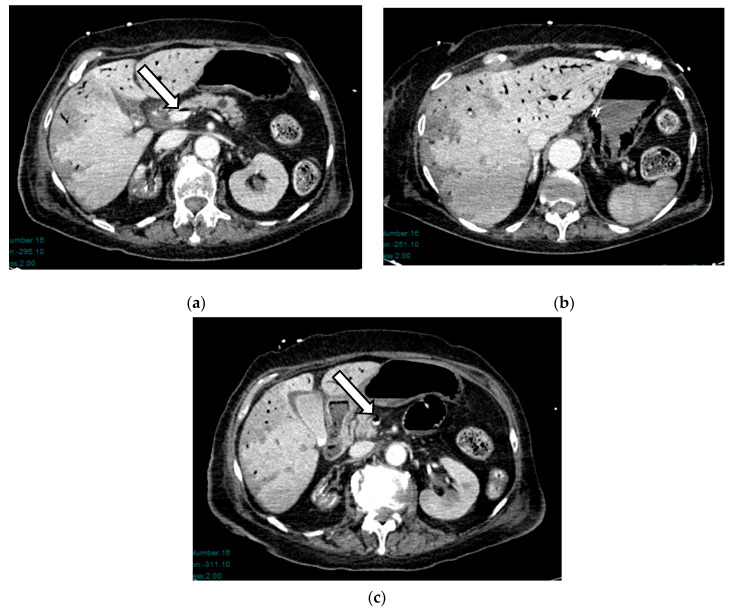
AMVI in an 84-year-old woman: axial contrast-enhanced CT scans show venous gas in the portal vein (arrow) and intrahepatic portal branches (a,b), as well as in the SMV (arrow) (c).
